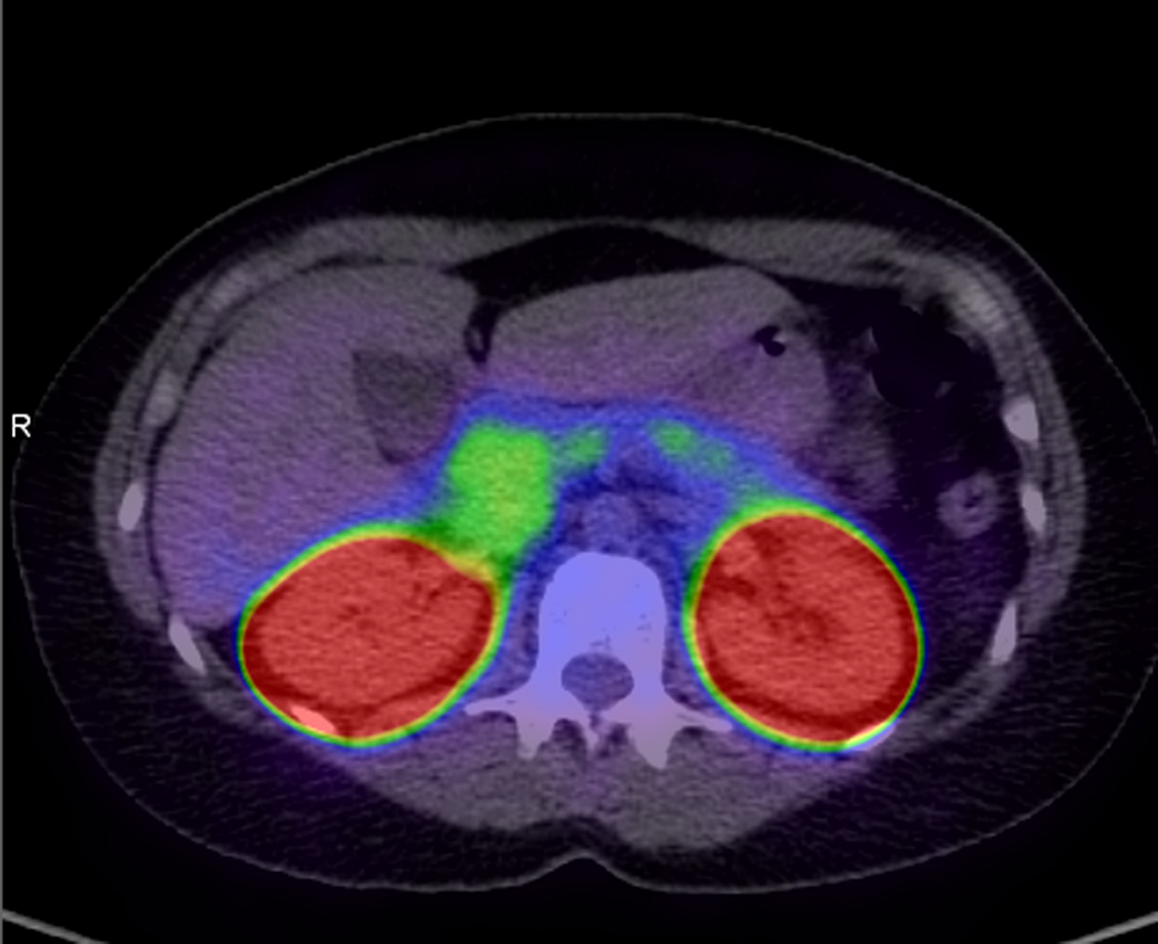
Ga-68 GLP-1 PET-CT demonstrating diffuse GLP-1 uptake in an enlarged pancreatic tail.
